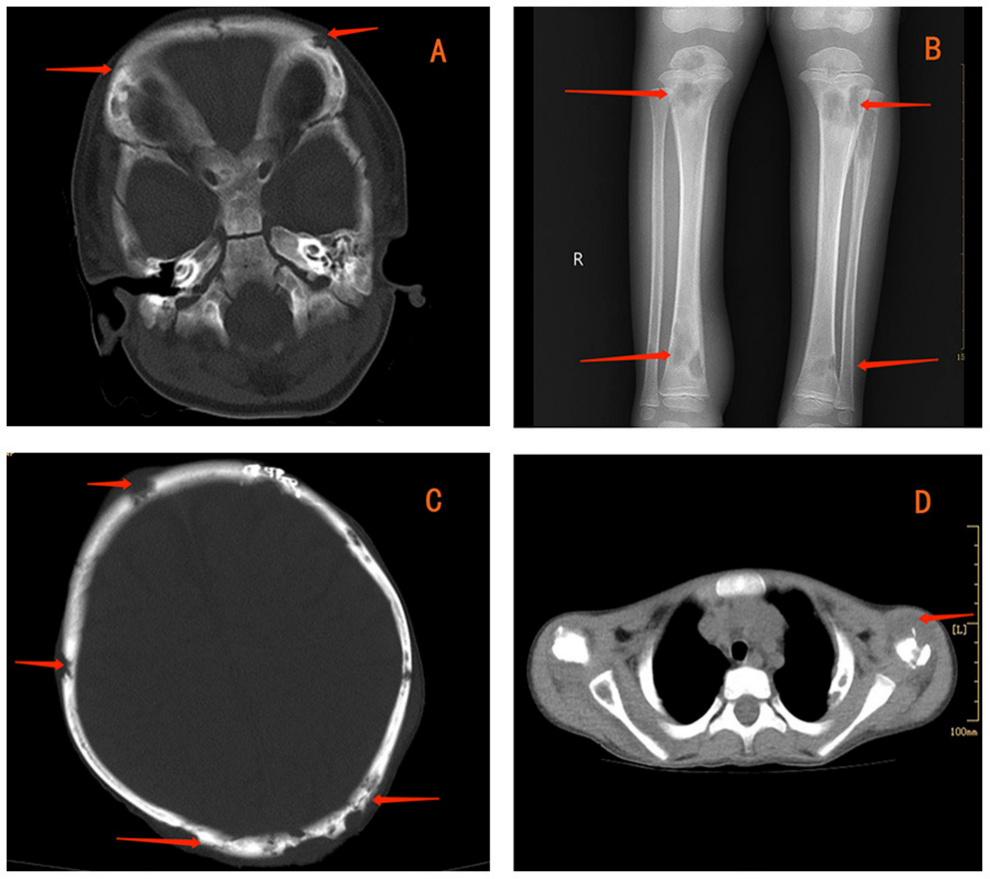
Contrast-enhanced computed tomography (CT) showing multiple lytic bone lesions in the skull (A). Radiography reveals multiple lytic bone lesions in bilateral tibias (B). CT indicates osteolytic lesions in the skull (C) and left clavicle (D).
